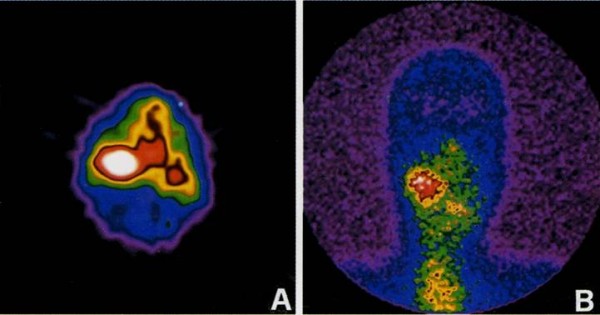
A) Markedly increased focal tracer uptake in the right cervical region in both planar and B) SPECT scans due to a massive chemodectoma at the right carotid bifurcation.
